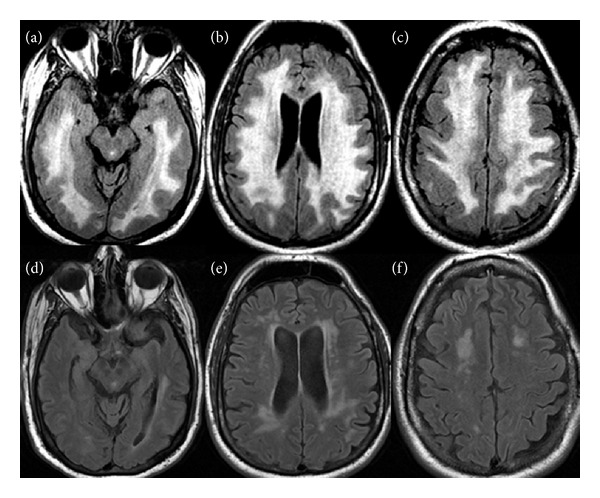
Magnetic resonance imaging findings in case 2 at initial presentation (upper row) and follow-up (lower row). (a)–(c) Axial FLAIR images, taken at the levels of the hippocampi, mid lateral ventricles, and centrum semiovale, demonstrate confluent areas of abnormal high signal in the deep white matter with sparing of the subcortical white matter and overlying cortex. (d)–(f) Axial FLAIR images, at similar levels to (a)–(c), demonstrate marked decrease in the abnormal signal in the cerebral white matter.
